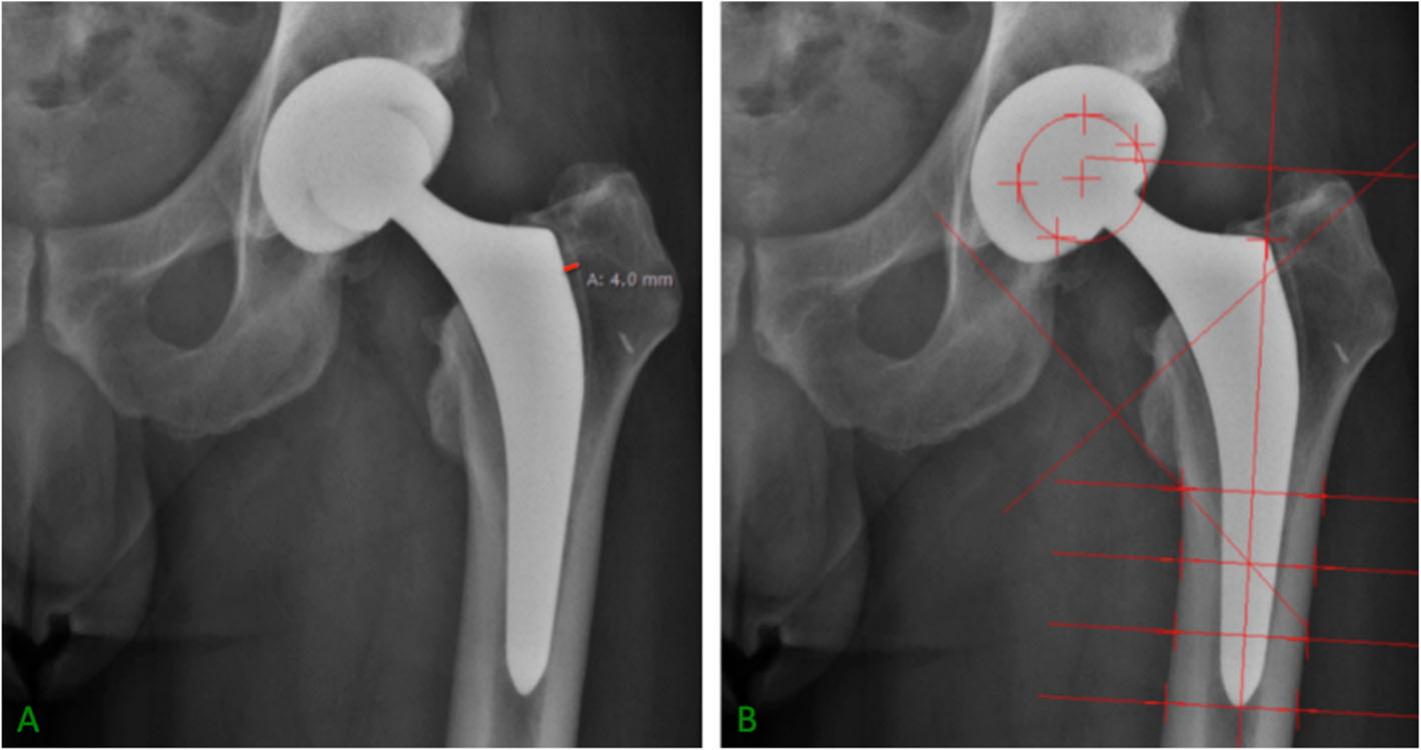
a Anterior-posterior radiograph showing a periprosthetic radiolucency of 4 mm in zone 7 of Gruen. b EBRA-FCA measurements on an anterior-posterior radiograph
